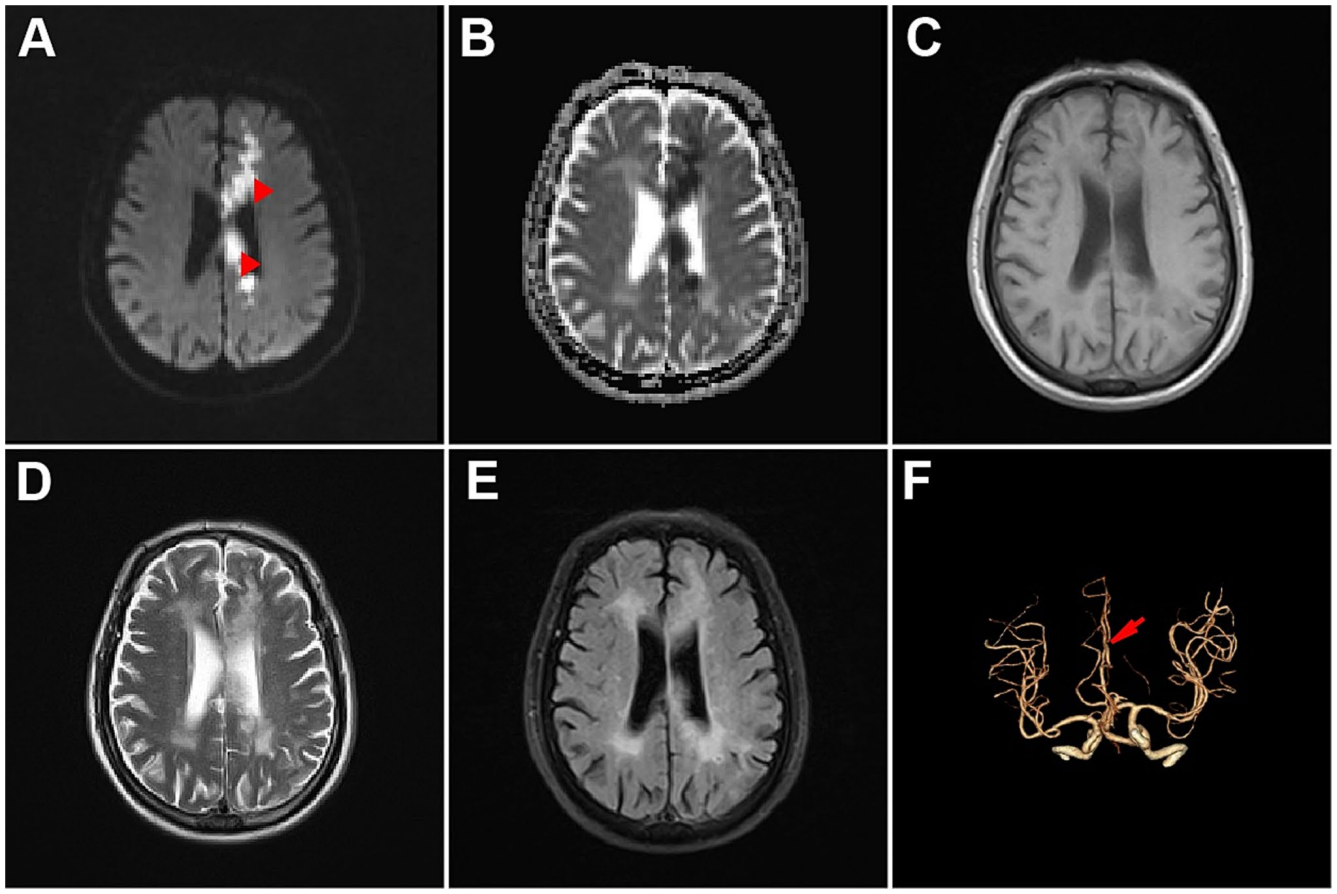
Brain imaging of the patient. (A) Diffusion-weighted image. (B) Apparent diffusion coefficient image. (C) T1-weighted image. (D) T2-weighted image. (E) Fluid attenuated inversion recovery image. (F) Brain computed tomography angiography image.
